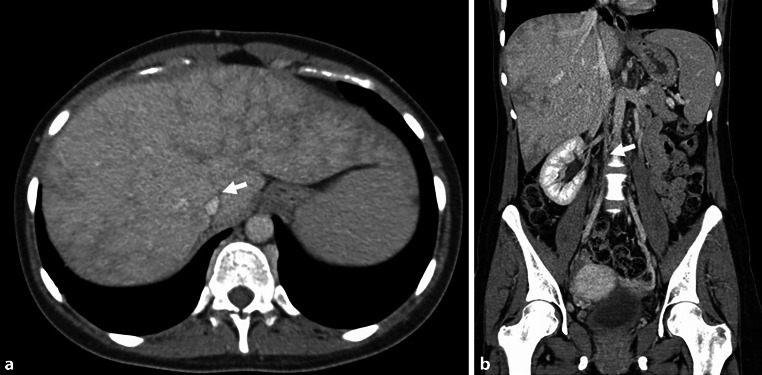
a The axial CT scan of the abdomen shows the Budd-Chiari syndrome with a thrombosis of the middle and left hepatic veins (*arrow*). b Coronal reformation of the CT shows a fibrotic occlusion of the inferior vena cava (*arrow*)
